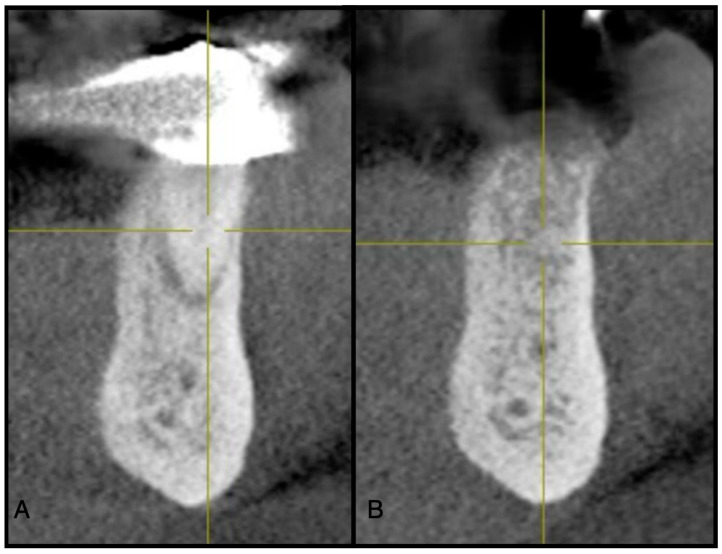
(A) Pre-operative CBCT at site #44. (B) Three-month post-operative radiograph of site #44 showing adequate ridge dimensions for implant placement after utilizing an e-PRF membrane over bone allograft particulate.
